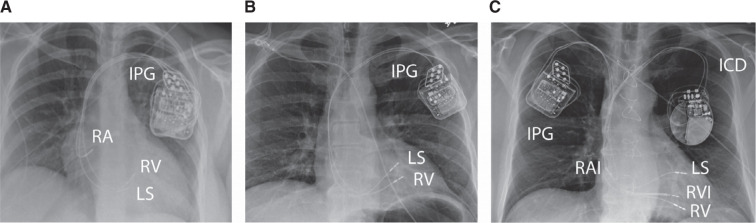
Chest radiograph of a cardiac contractility modulation (CCM) implant. Displayed are (A) a 3-lead CCM system only, (B) a 2-lead CCM system only, and (C) a 2-lead CCM system with a dual-chamber implantable cardioverter-defibrillator. Of note, the designation of the septal CCM leads as “LS” and “RV” is not determined by location, but rather the differential timing of sensing on each lead at implant. *Abbreviations:* CCM, cardiac contractility modulation; ICD, implantable cardioverter-defibrillator; IPG, implanted pulse generator; LS, local sense; RA, right atrial; RAI, right atrial ICD; RV, right ventricular; RVI, right ventricular implantable cardioverter-defibrillator.
